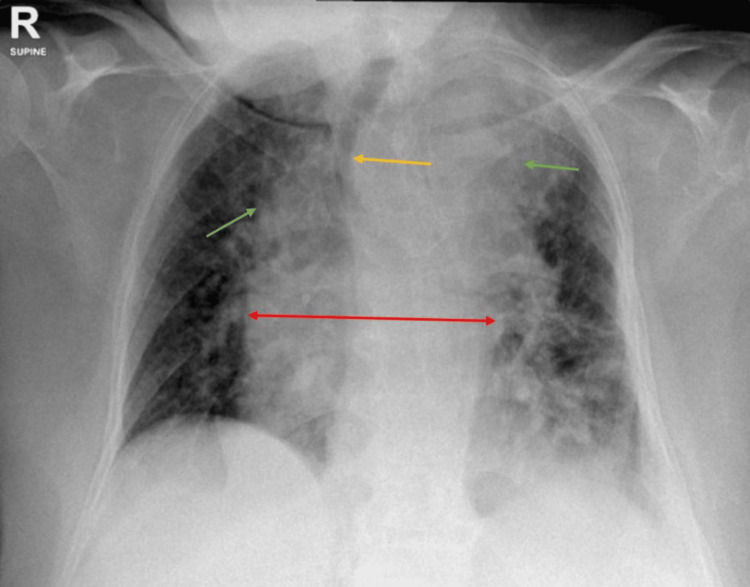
Chest X-ray taken on day 4 post-admission, showing worsening bilateral upper lobe consolidation (green arrows) with mediastinal widening (red arrow) and trachea significantly shifted to the right side (yellow arrow)
